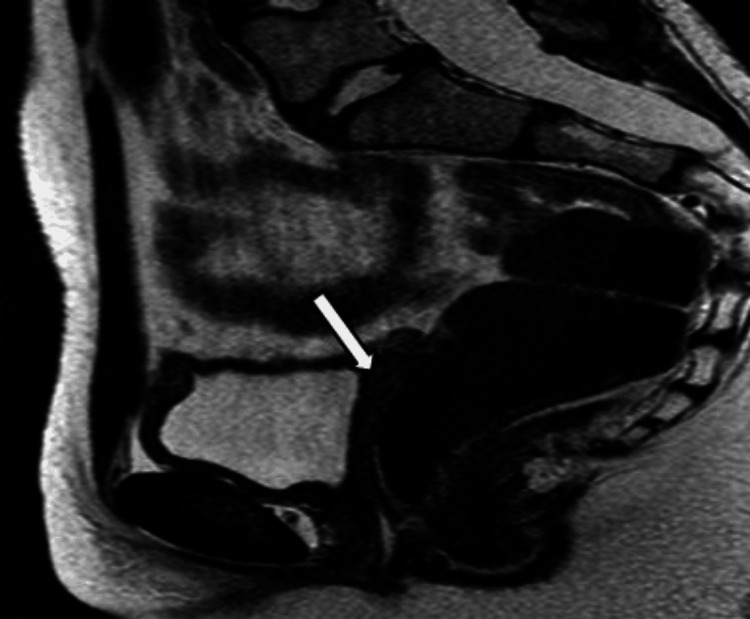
T2-weighted MRI of the pelvis. The sagittal view (in the younger sister) showing the rudimentary uterus (the white-shaded arrow).
